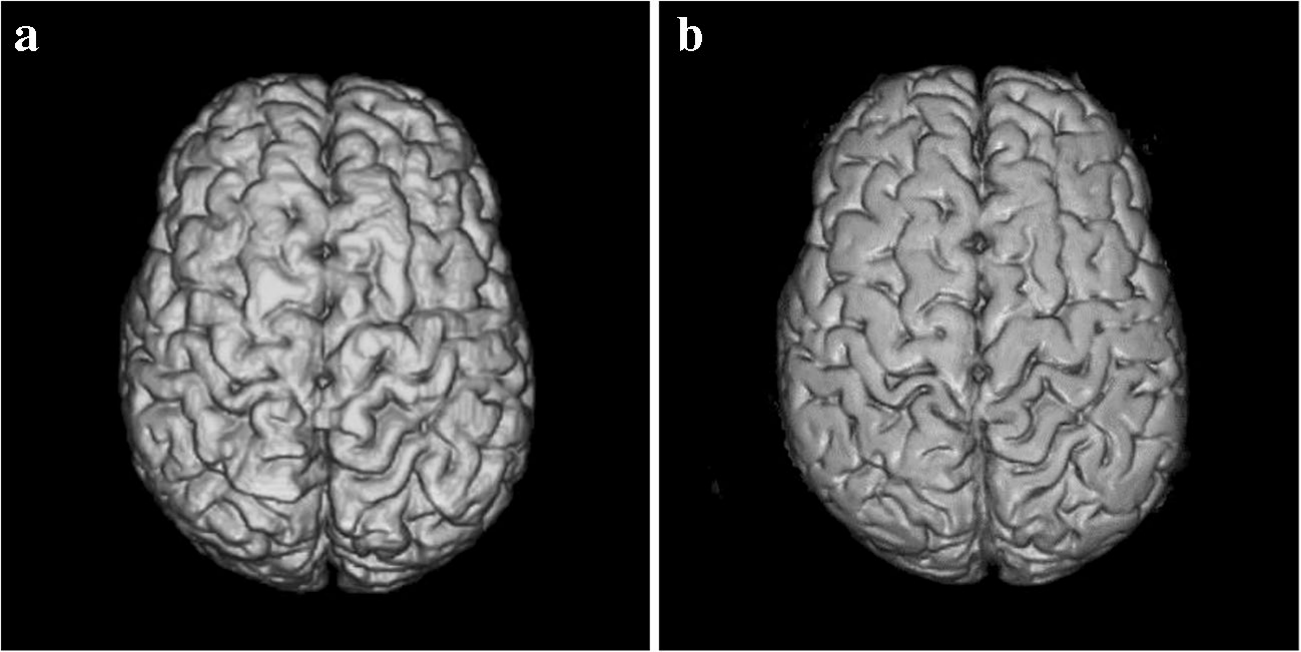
A 5-year-and-8-month-old girl with a normal magnetic resonance imaging brain scan. a, b Two successful segmentations produced using the Computational Anatomy Toolbox version 12 segmentation method in the 3-dimensional Standard Tessellation Language. The volume model (a) is visually higher in quality and demonstrates more cortical gyral and sulcal detail than the volume model (b) which shows flattened and less defined gyri. Gyral and sulcal detail is an important component of brain surface demonstration
